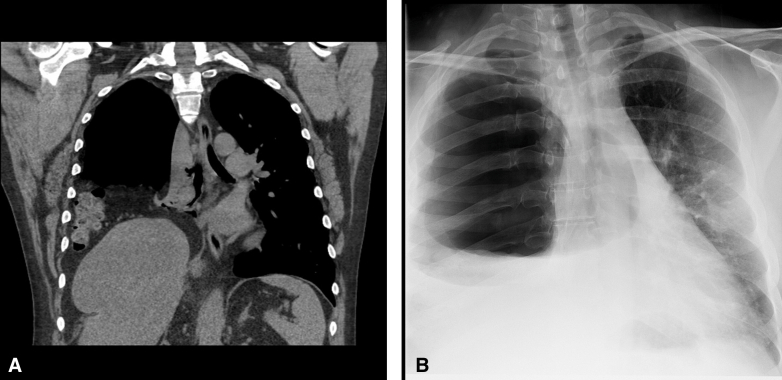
A, Computed tomography of the chest showing a large right-sided diaphragmatic hernia. B, Radiograph of the chest demonstrating right-sided bulla with mediastinal shift.
